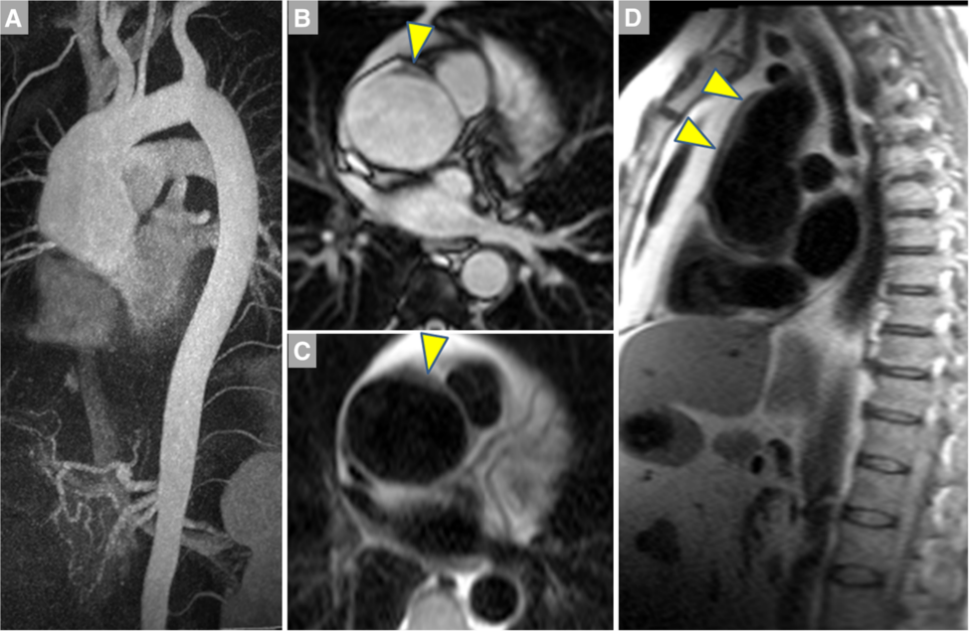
A 58 year-old male with fatigue and palpitations underwent transthoracic echocardiography that indicated dilatation of the aortic root. CMR was ordered to assess the aorta. A. MIP of the CE-MRA shows marked dilatation of the ascending aorta, which measured up to 6 cm in diameter compared to the 2.5 cm arch. B. Single heartbeat true FISP bright blood image shows thickening of the aortic wall (arrowhead), also evident on HASTE dark blood imaging in the axial (C) and sagittal planes (D).
